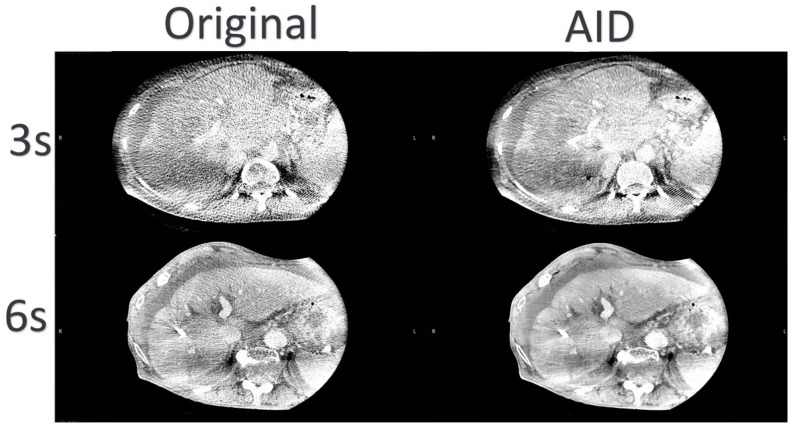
Representative TIPSS-CBCT Images: Noticeable Image Quality Enhancement with Minimized Noise and Motion Artifacts in the AID Series, Resulting in Superior Definition of the Portal vein.
